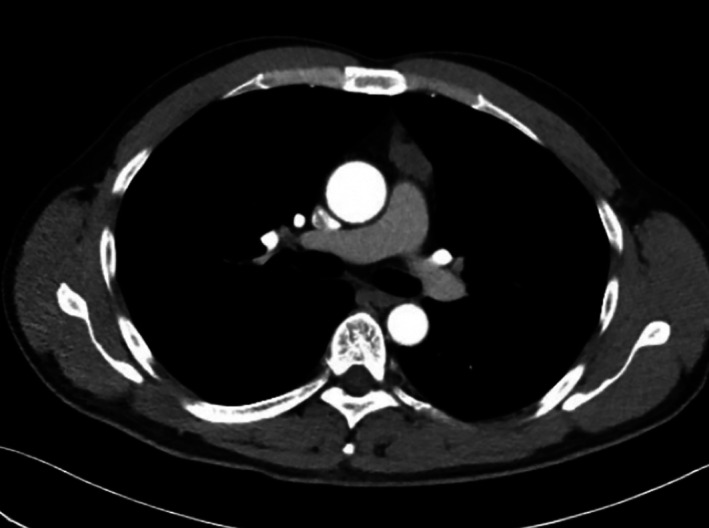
Chest contrast‐enhanced computed tomographic scan in the coronal view shows a soft‐tissue mass located in the anterior mediastinal
